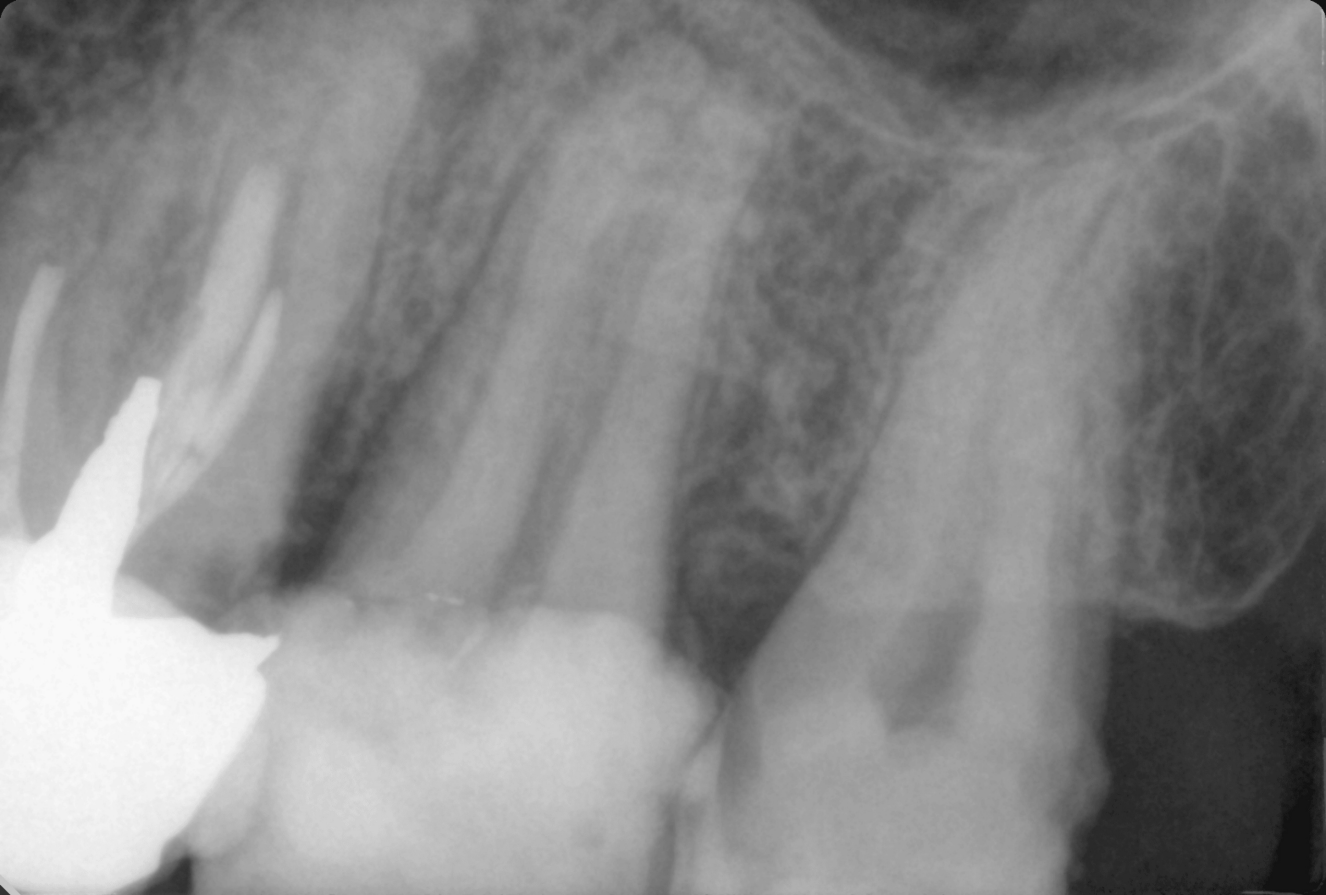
Preoperative radiograph of tooth 27
